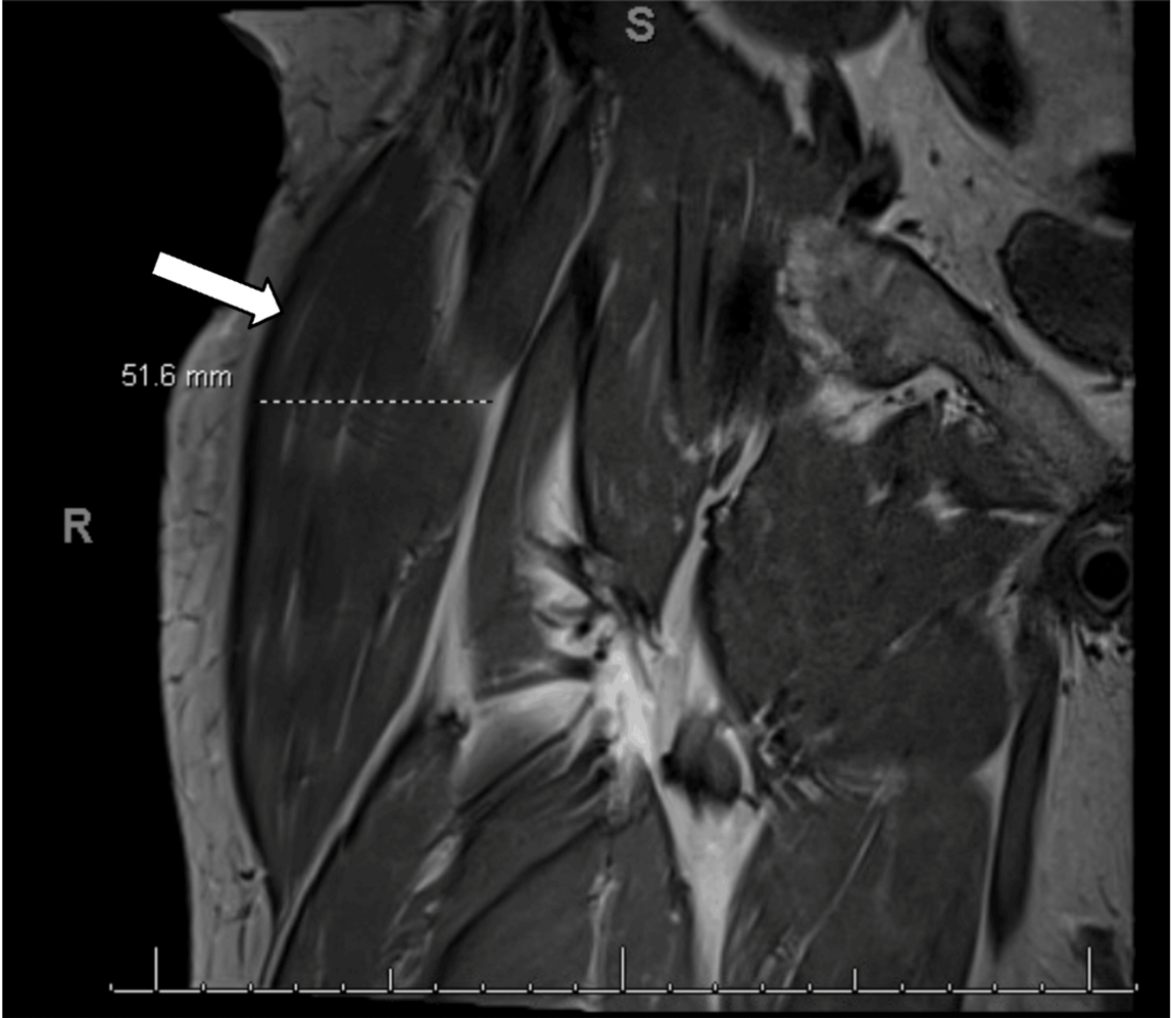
MRI showing hypertrophy of the TFL The scan of the right lower extremity in the coronal plane shows an enlarged TFL muscle measuring 51.6 mm as demarcated in the image (white arrow). TFL, tensor fascia lata; MRI, magnetic resonance imaging
